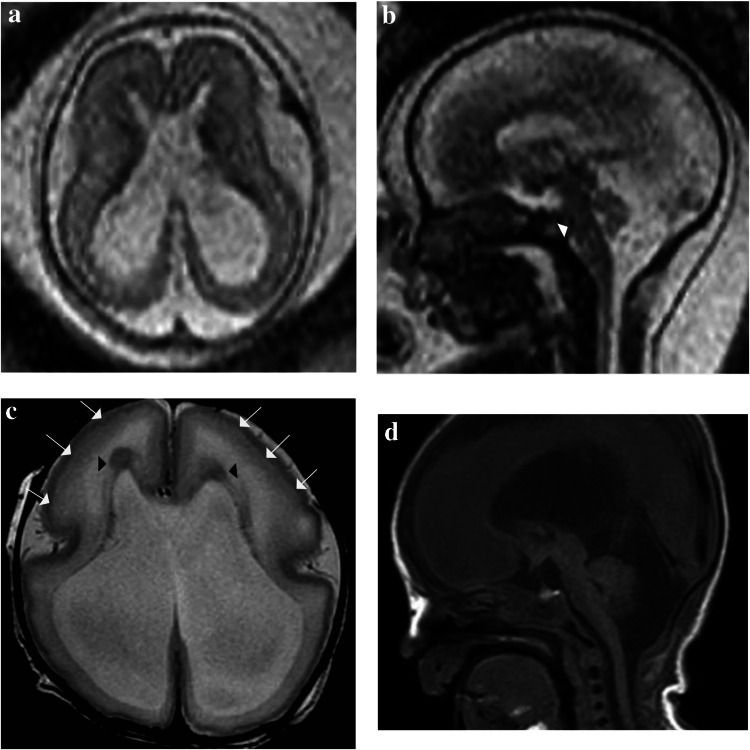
Vermian hypoplasia, brainstem hypoplasia, and lissencephaly. Fetal MRI axial supratentorial T2 single-shot fast-spin echo (a) performed at 22 weeks shows ventriculomegaly and a slightly shallow appearance of Sylvian fissures for age. Sagittal midline image (b) shows a small vermis and a flattened pons (*white arrowhead*). Postnatal MRI performed at 5 days of age shows lissencephaly. Axial T2 (c) sequence through the supratentorial brain shows severe ventriculomegaly, absent cerebral sulci aside from Sylvian fissures, an abnormal band of T2-isointense heterotopia in the bifrontal subcortical white matter (*white arrows*), and bifrontal periventricular heterotopic tissue (*black arrowheads*). Sagittal T1 (d) sequence through the midline brain redemonstrates the pontine and mild vermian hypoplasia seen on fetal MRI
